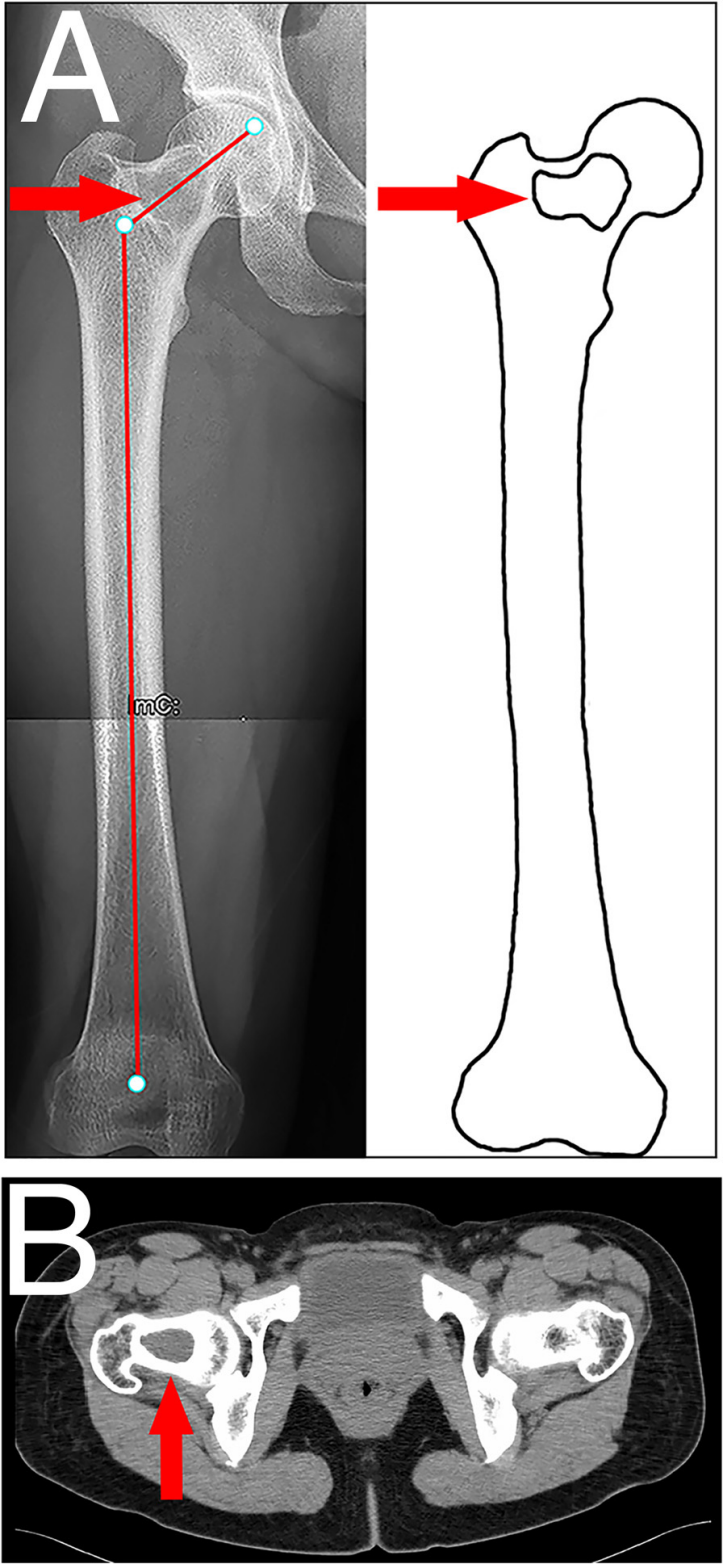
Type 1 femur in a 43-year-old woman. The *red arrow* indicates the lesion. a Fibrous dysplasia was present in the femoral neck. The neck-shaft angle was normal (125°). There was no varus deformity in the proximal femoral shaft. b The cortex around the lesion was intact on axial CT
